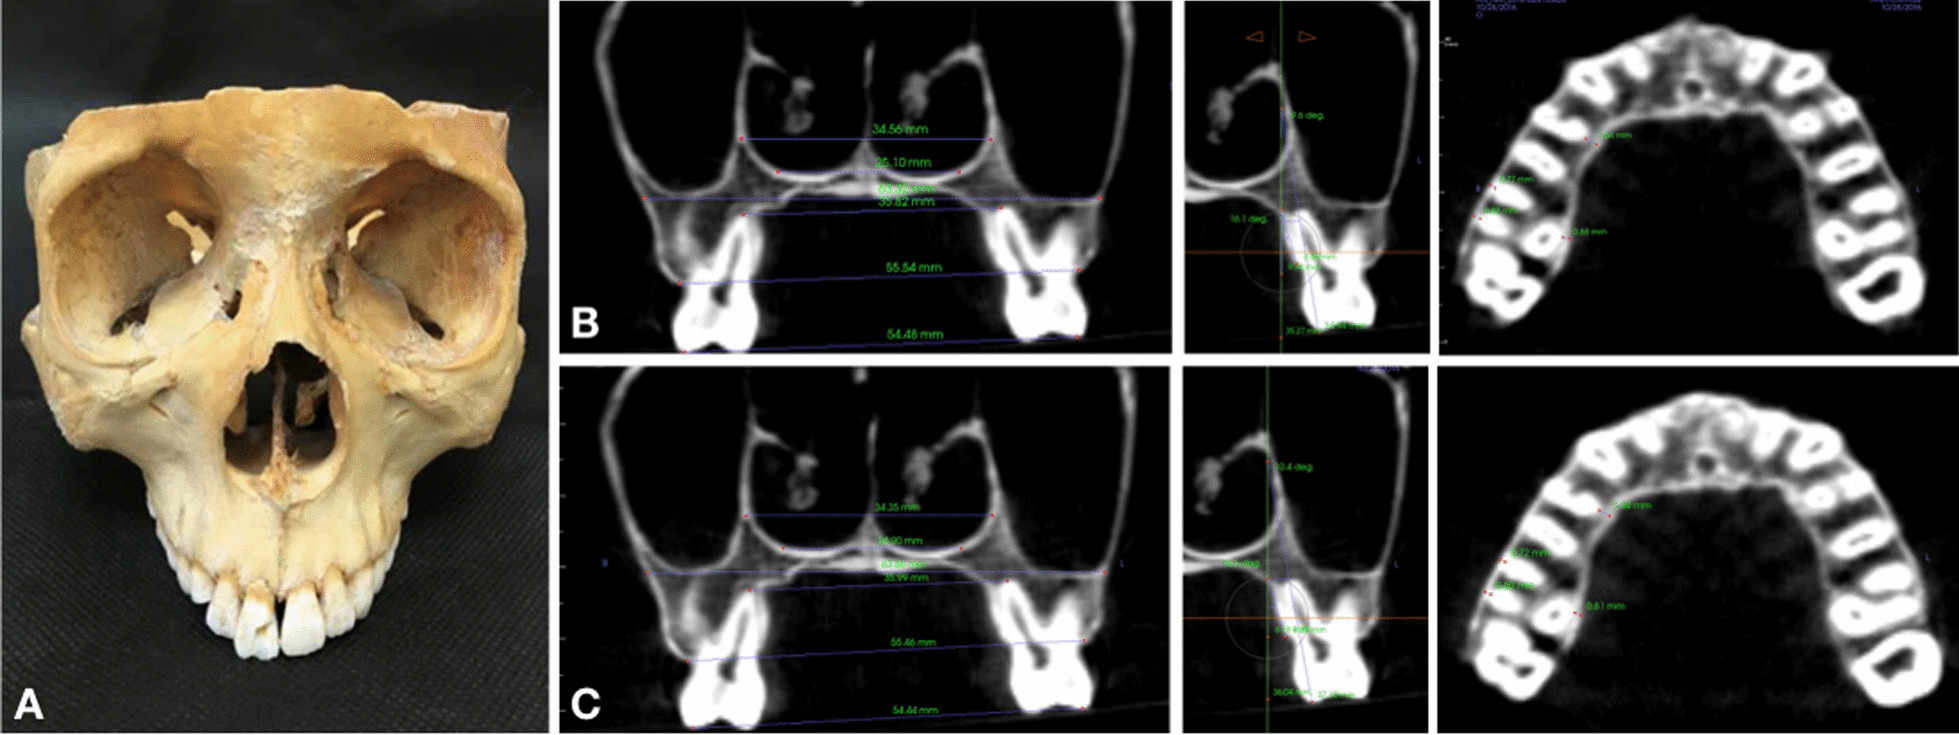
Preliminary study. a Human dry skull; b CBCT images in standard mode; c CBCT images in low-dose mode
